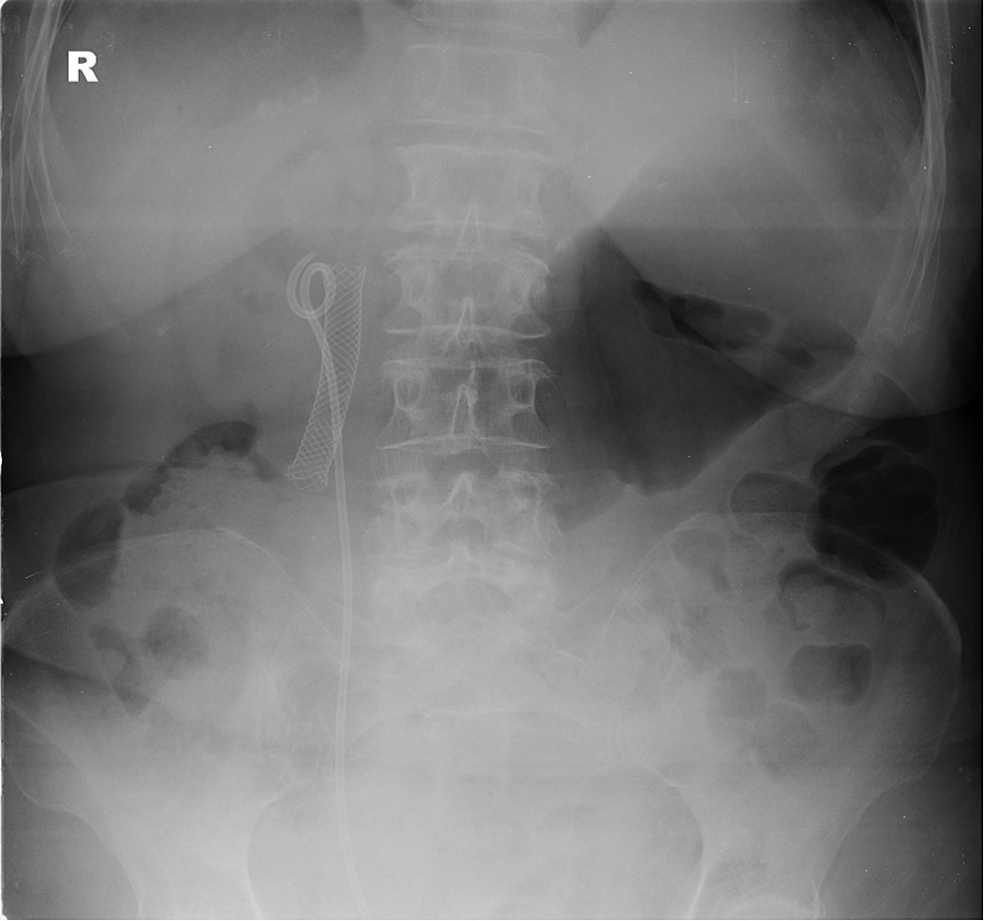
Abdominal radiograph on admission
